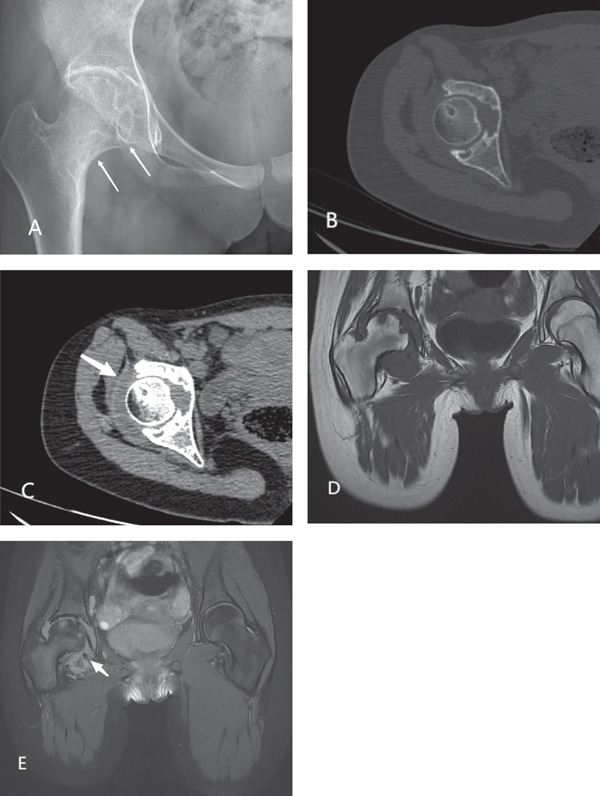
Pelvic imaging of the right hip joint of a 14‐year‐old girl with right hip pain and limited mobility. (A) X‐ray imaging reveals a bone defect in the right femoral head, femoral neck, and right hip joint, with a thin sclerotic edge at the boundary with normal bone. (B) Computed tomography imaging shows bone destruction at the joint edge, with a visible sclerotic zone at the junction with normal bone. (C) The synovial membrane around the right hip joint is unevenly thickened, presenting as a low‐density circular shadow surrounding the joint. (D) Magnetic resonance imaging shows thickening of the synovial membrane of the right hip joint with irregular edges, as well as low signal intensity on T1‐weighted images and high signal intensity on PDWI+FS images. (E) Small nodules with low signal intensity and clear boundaries are visible on T1‐weighted and T2 fat‐saturated images.
